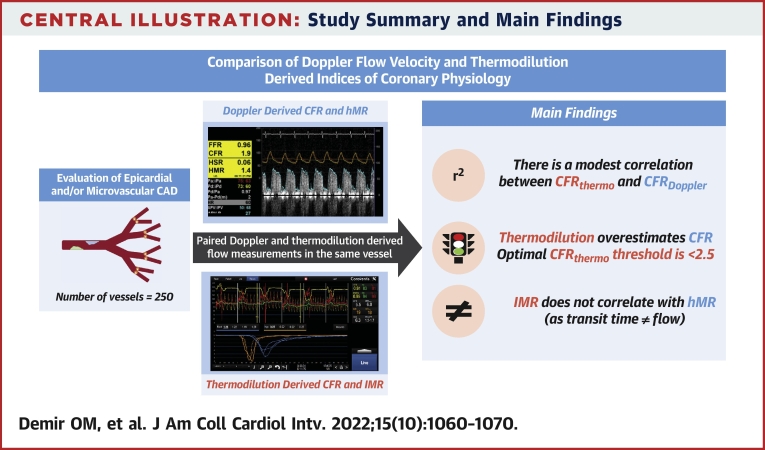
Study Summary and Main Findings CFR = coronary flow reserve; CFR_Doppler_ = Doppler-derived coronary flow reserve; CFR_thermo_ = thermodilution-derived coronary flow reserve; hMR = hyperemic microvascular resistance; IMR = index of microvascular resistance.
